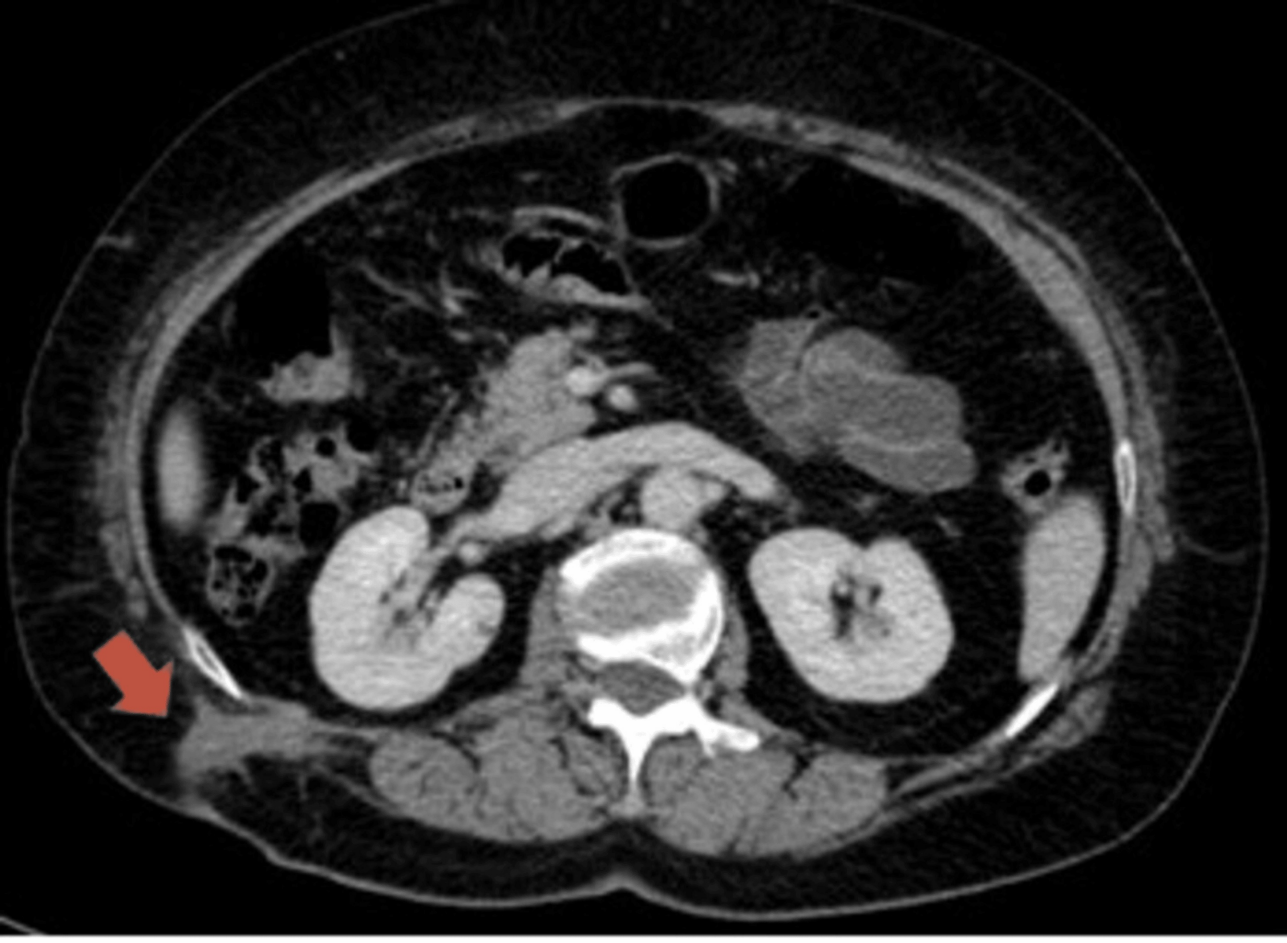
Axial CT image shows an 11-mm thick tubular densification extending to the hepatorenal space to the abdominal wall (arrow), within which small calcic foci were identified that drained spontaneously through the skin solution.
